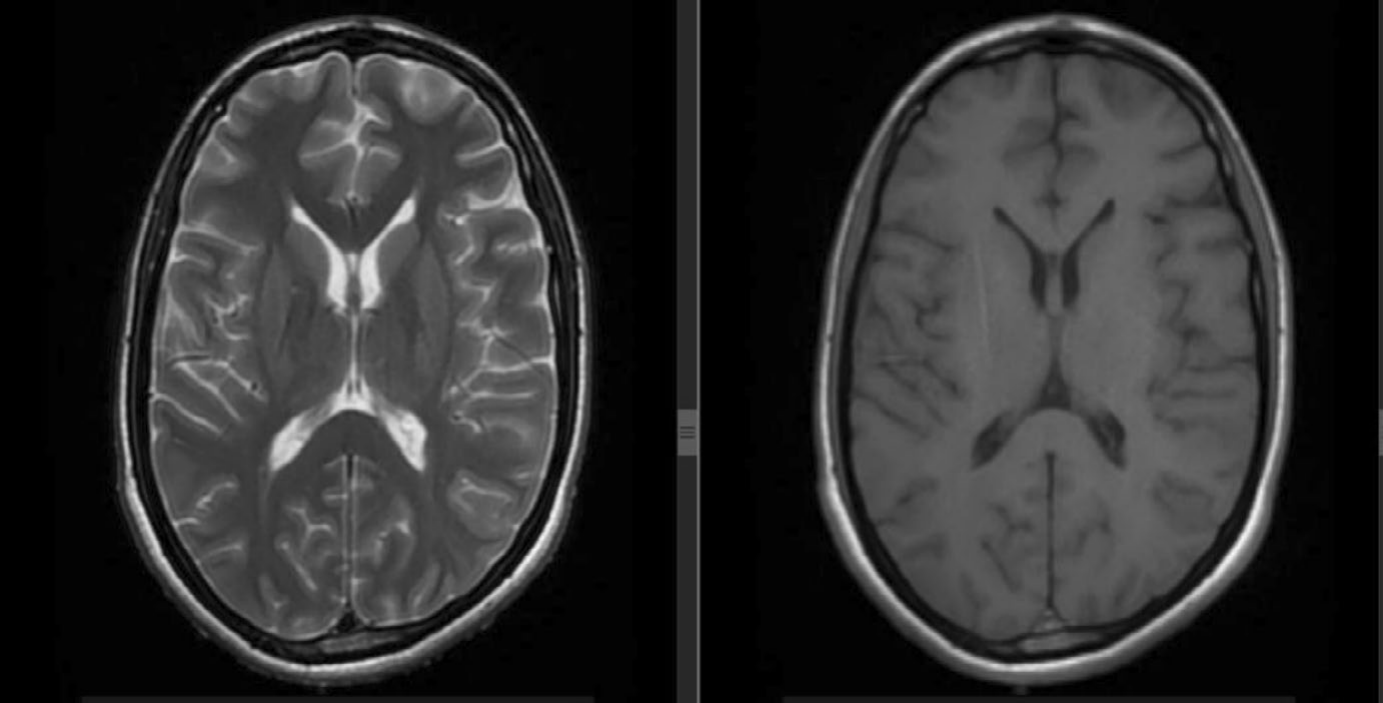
T1 weighted (right) and T2 weighted (left) brain MRI (normal). MRI, magnetic resonance imaging.
